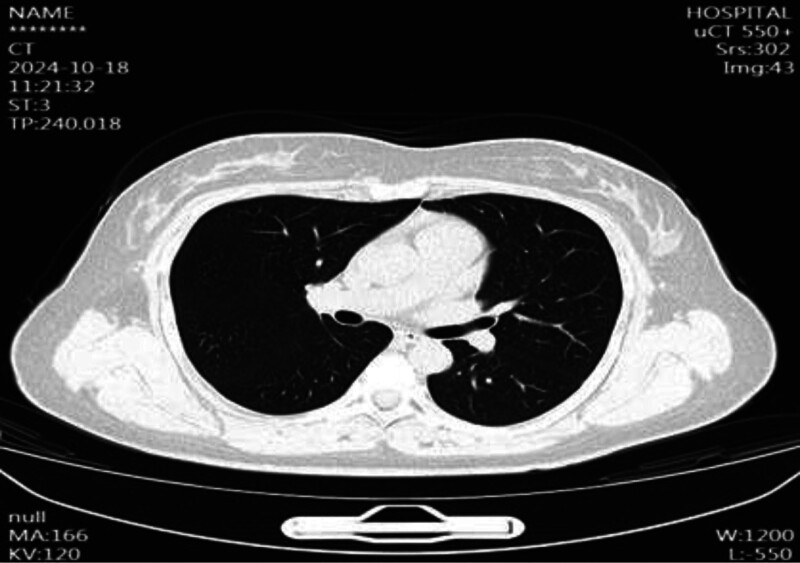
CT showing no significant abnormalities. CT = computed tomography.
